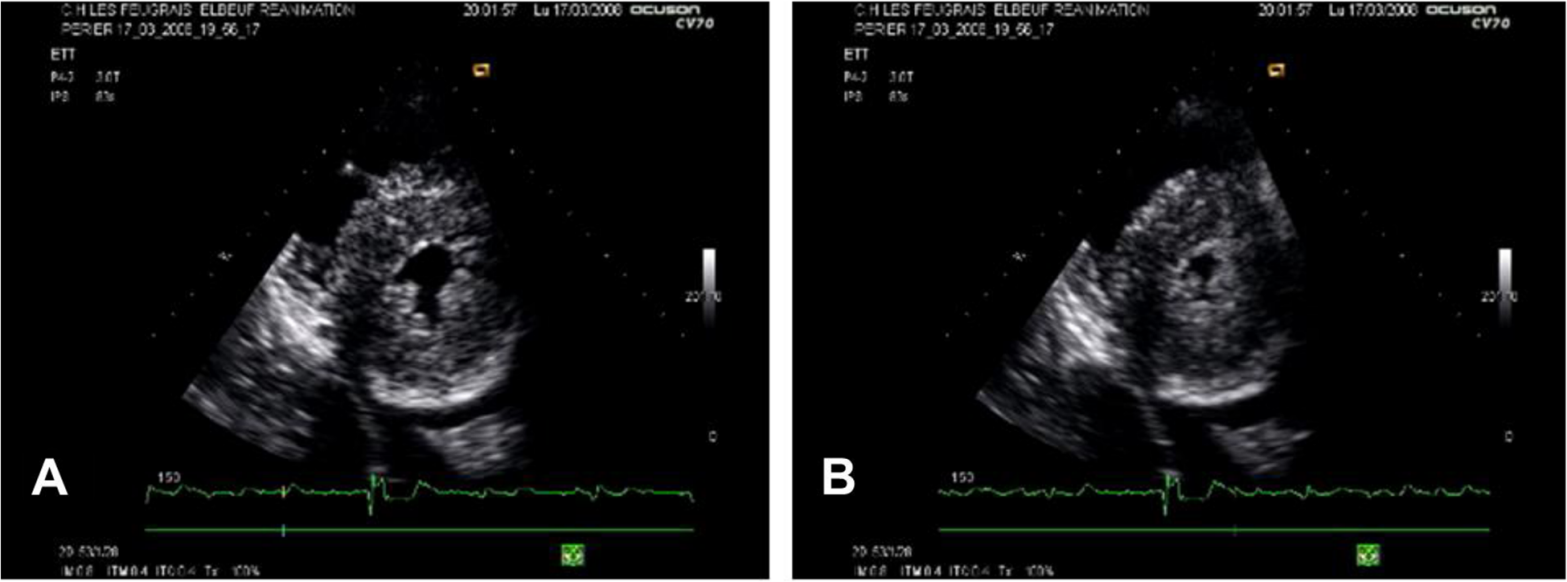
Pseudohypertrophy. Echocardiography in the parasternal short axis view. Ventricular wall appear thickened in these two-dimensional images, despite LV mass being unchanged. a The reduction in LVEDD size of the left ventricle (LV). b The near obliteration of the ventricular lumen in systole (“kissing” walls)
